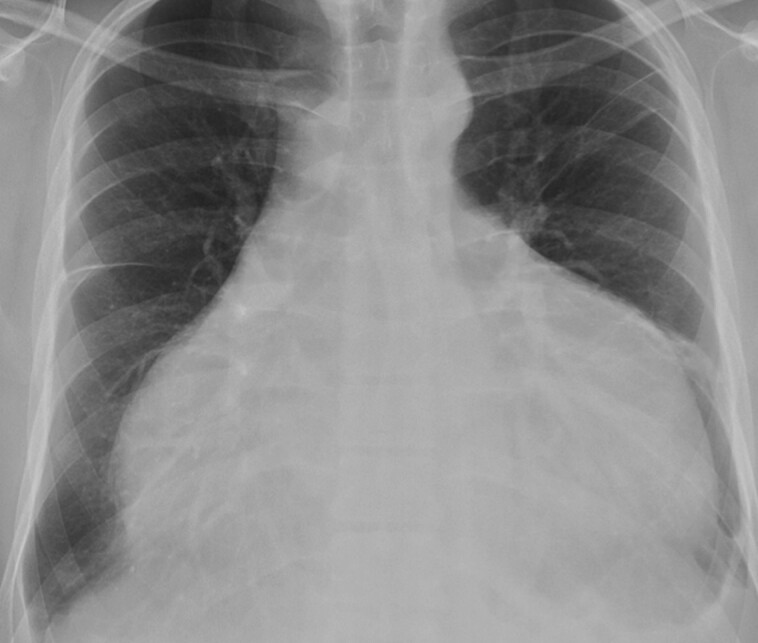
Posteroanterior chest X-ray, note the significantly enlarged cardiac silhouette.
